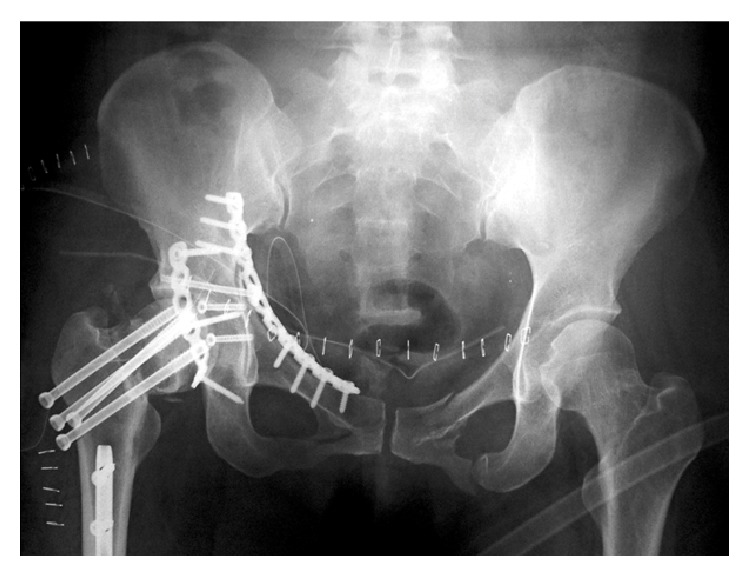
A postoperative radiograph of the acetabulum. Anterior and posterior plate fixation is performed.
